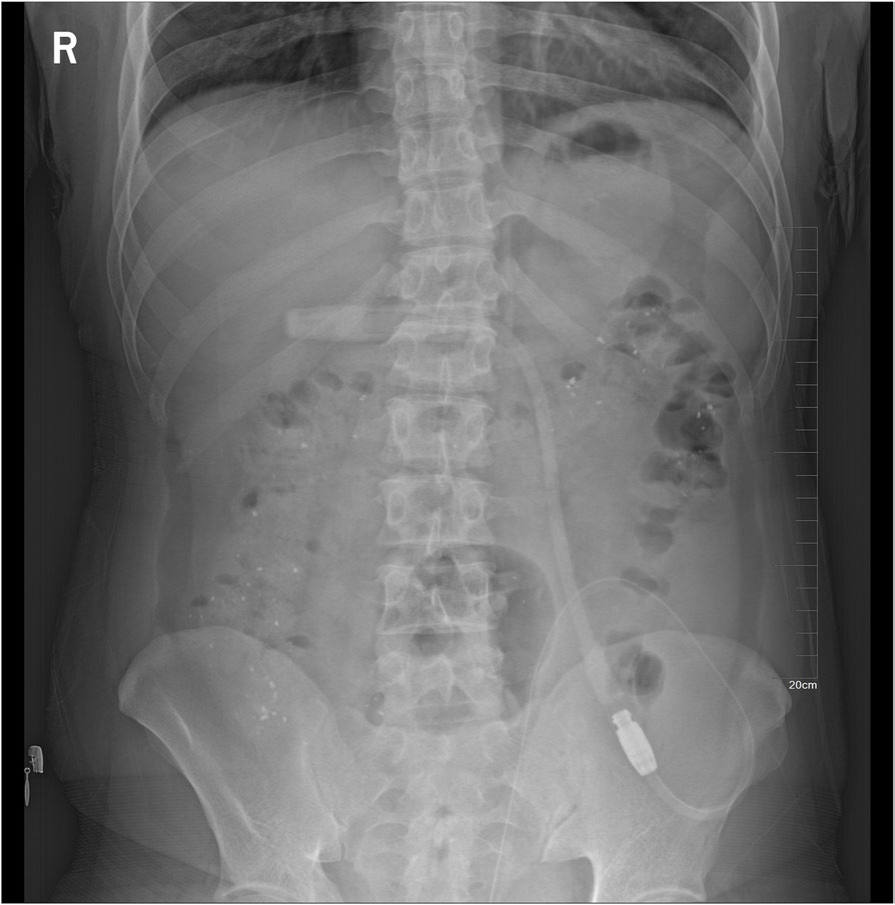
Abdominal X-ray of the patient
